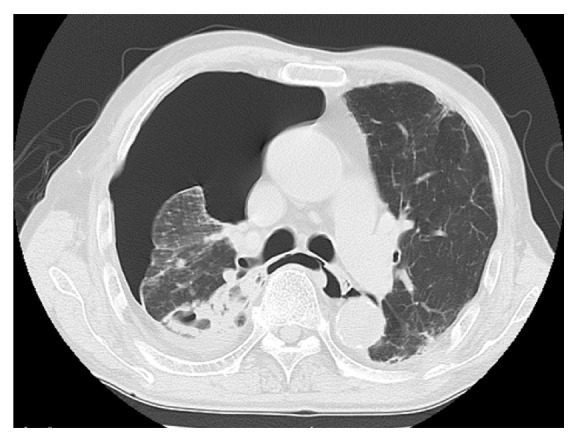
Chest computed tomography scan shows pneumothorax and pleural effusion in the right pleural cavity and a cavity lesion in the right upper lobe.
